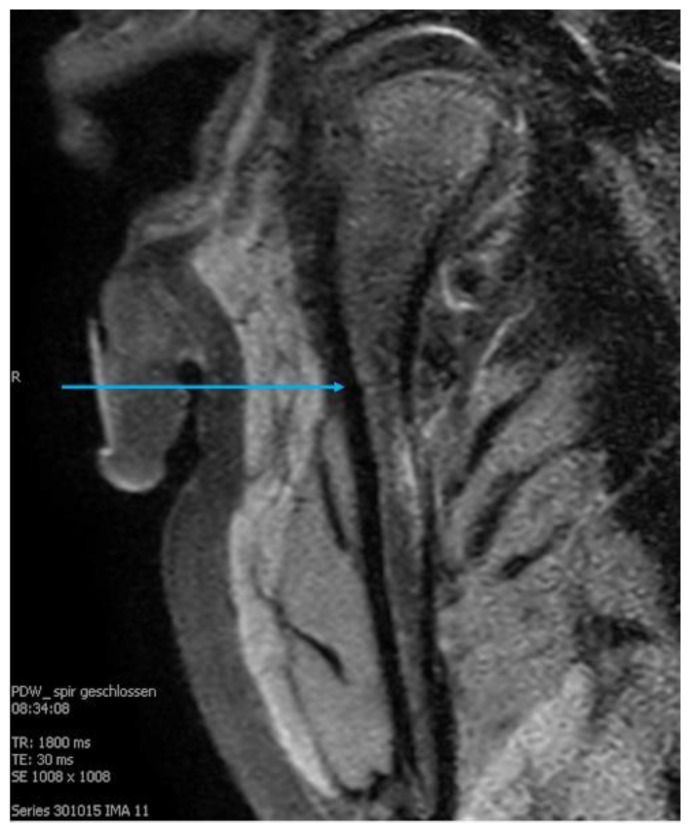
Magnetic resonance image of a mandibular condyle during follow-up (2). A line in the sense of a bone scar between the former fracture fragments is visible (blue arrow).
